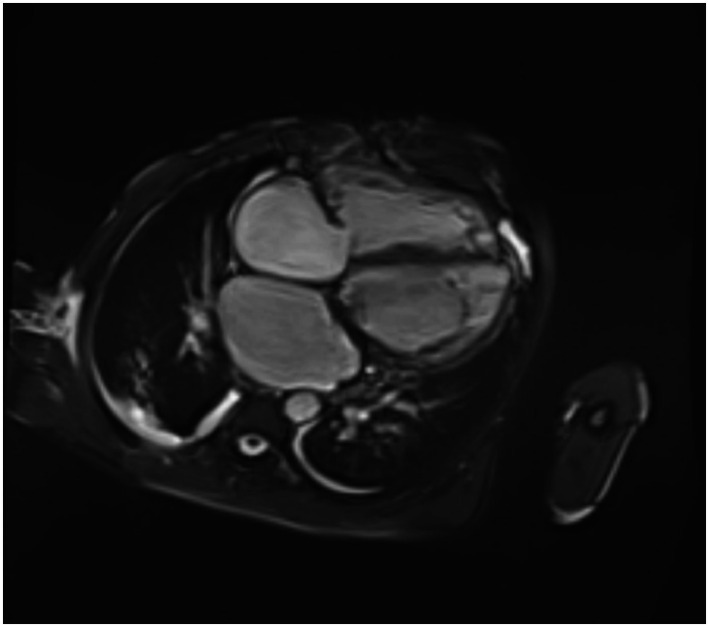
Cardiac MRI (short-axis cine sequence) showing severe biventricular dilatation and markedly reduced systolic function, with disproportionate right ventricular involvement, reflecting advanced biventricular failure in the context of prosthetic valve endocarditis.
